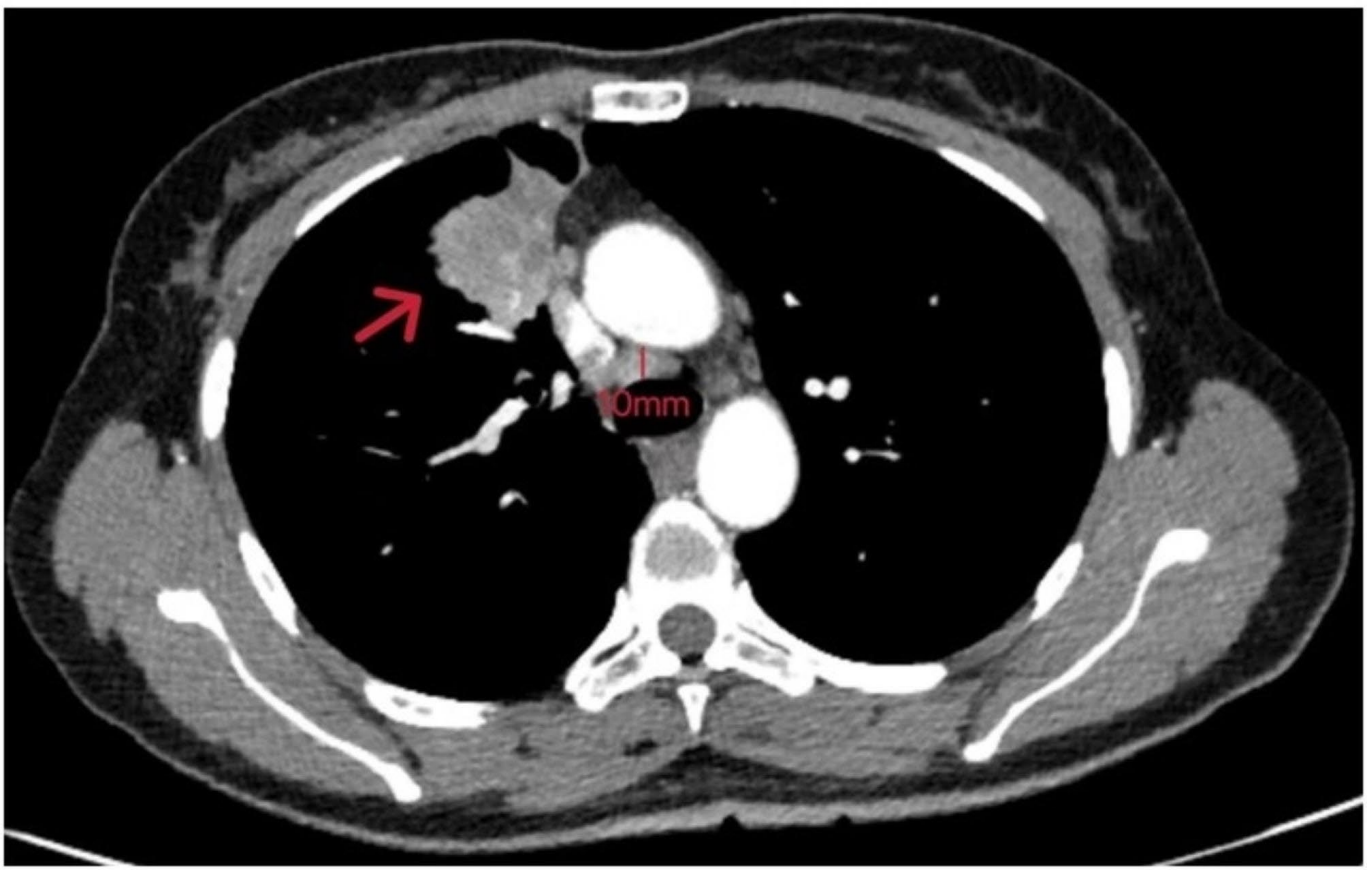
Enhanced contrast CT elucidated that statin 4R lymph node was slightly enlarged with 10mm short axis
